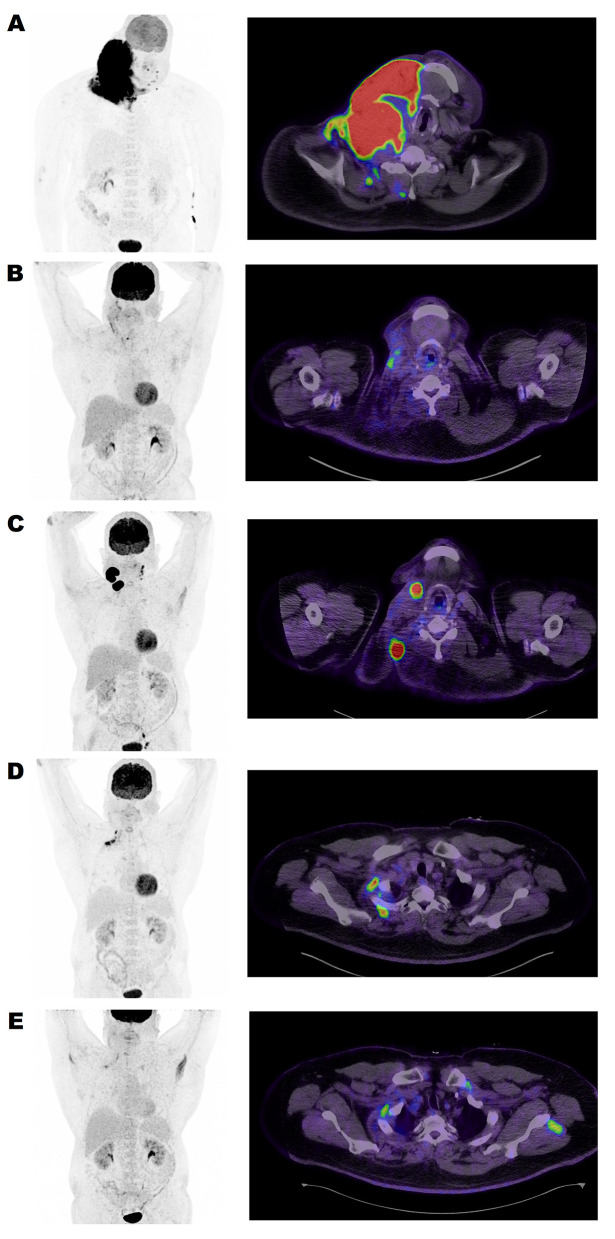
FDG PET/CT maximum intensity projection (left column) and fused PET and CT transaxial images (right column) from the lower neck region.
